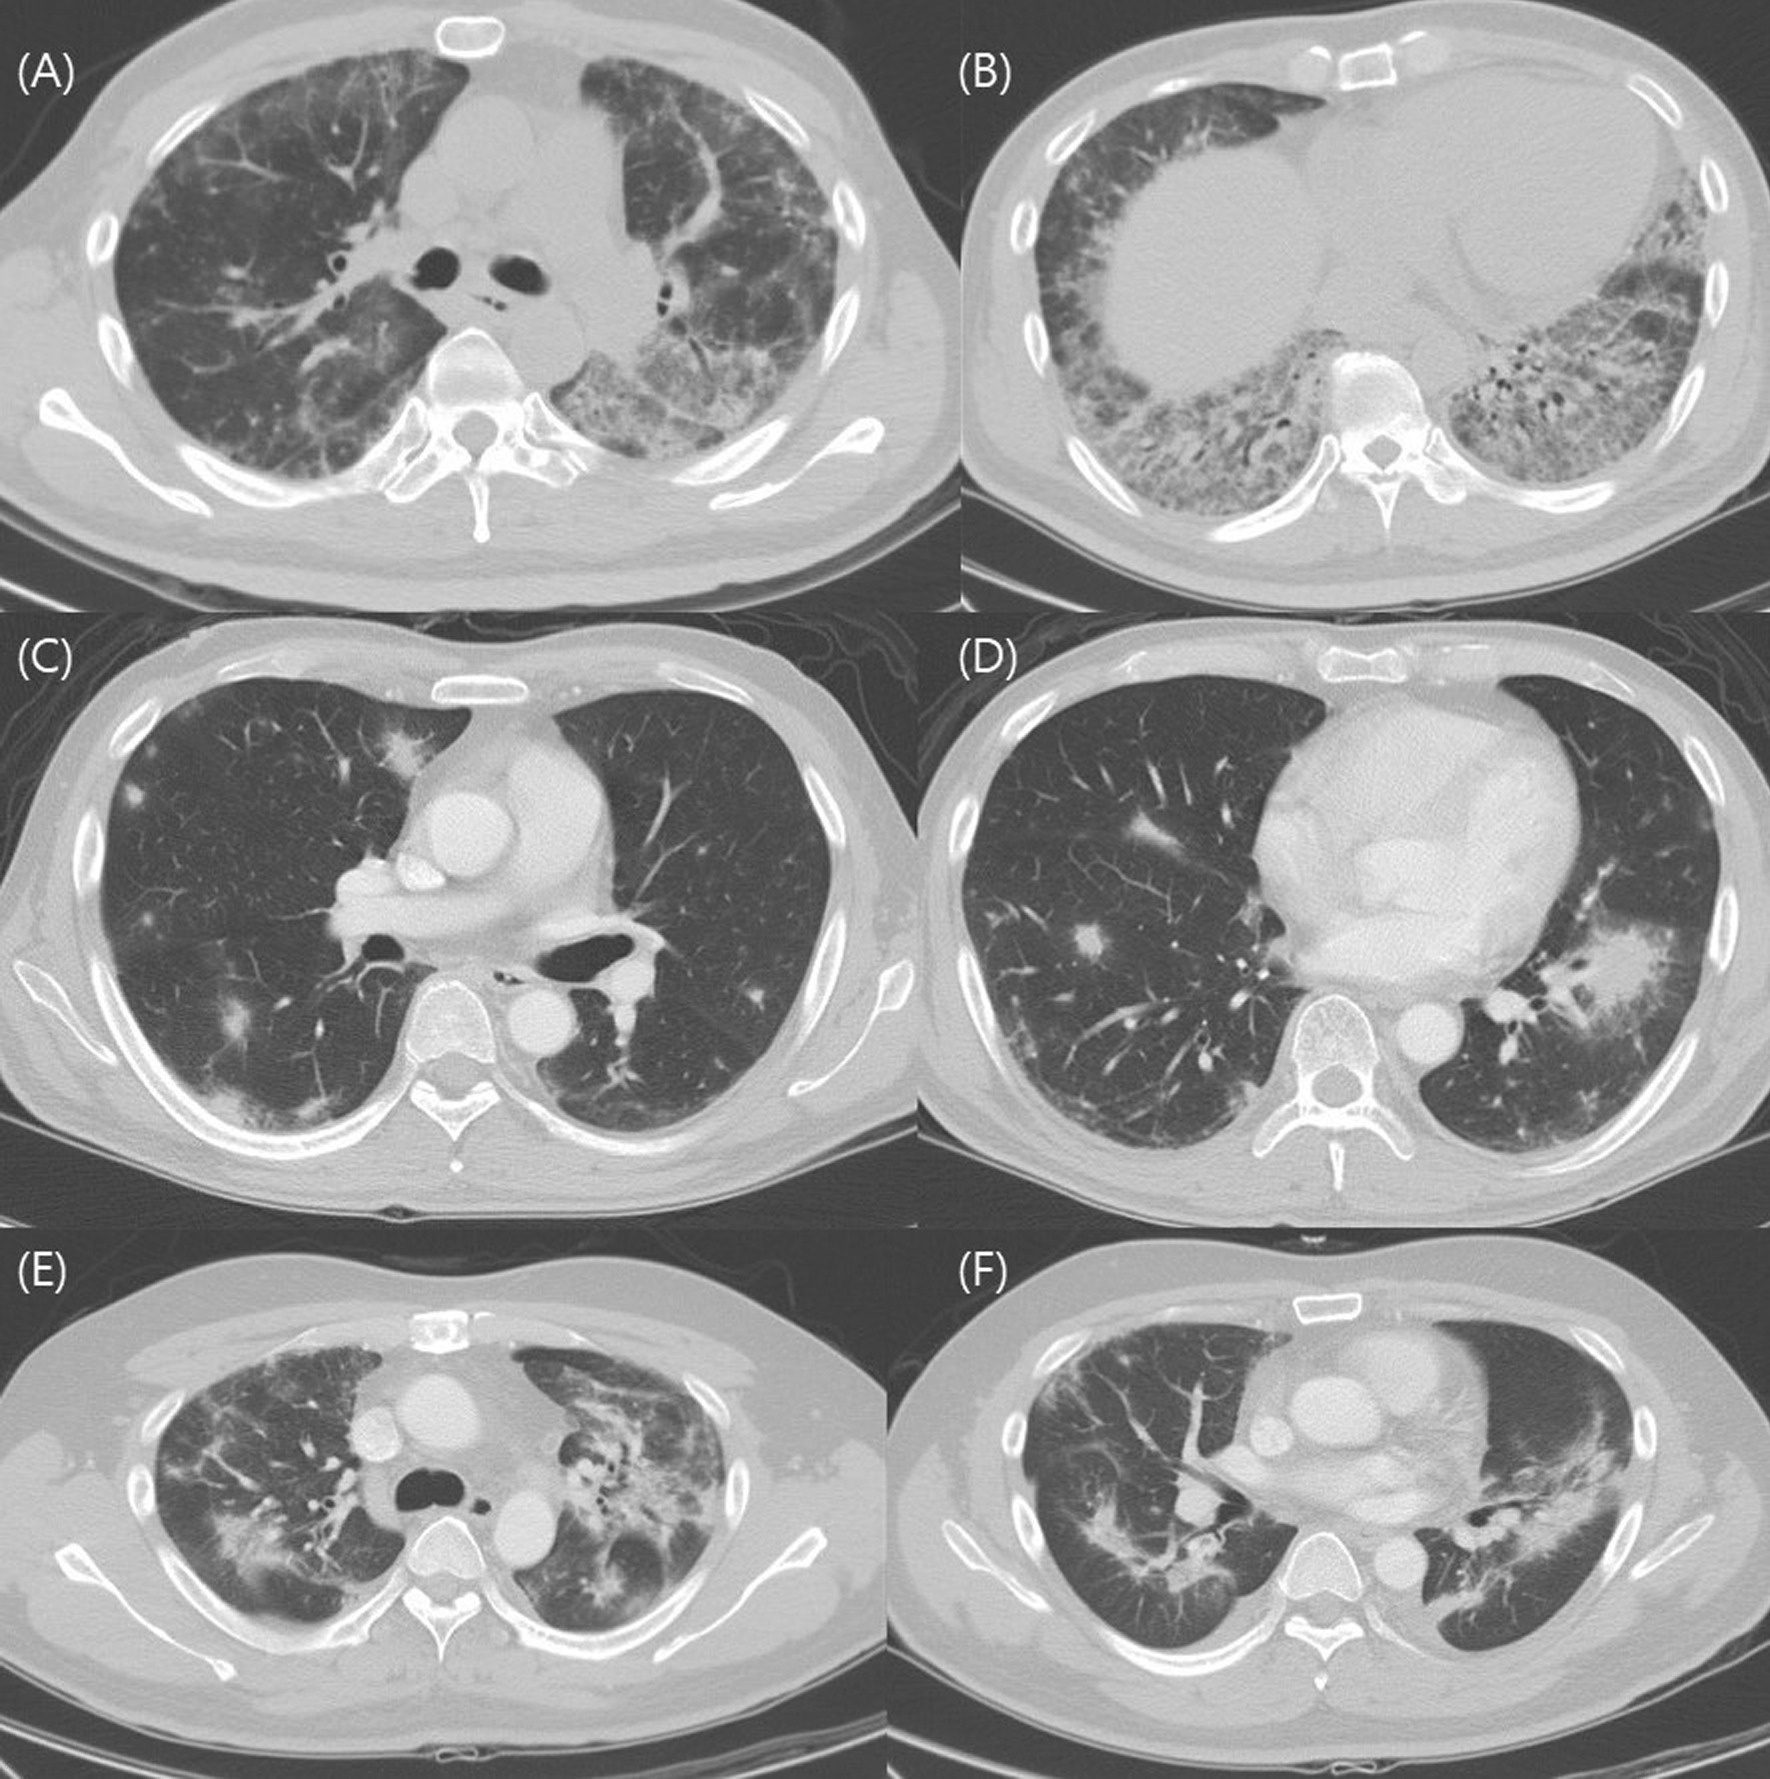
Representative CT images of acute fibrinous organizing pneumonia (AFOP). CT images A and B are from a 45-year-old man which show patchy and diffuse ground-glass opacity(GGO), peribronchovascular consolidation (GGO/consolidation dominant type). CT image C and D are from a 52-year-old man and show ill-defined nodular opacities in both lungs (nodule dominant type). CT image E and F are from a 40-year-old man and show fibrotic band at both lungs with diffuse GGO/consolidation (fibrosis dominant type). In figure F, small amount of bilateral effusion is seen
